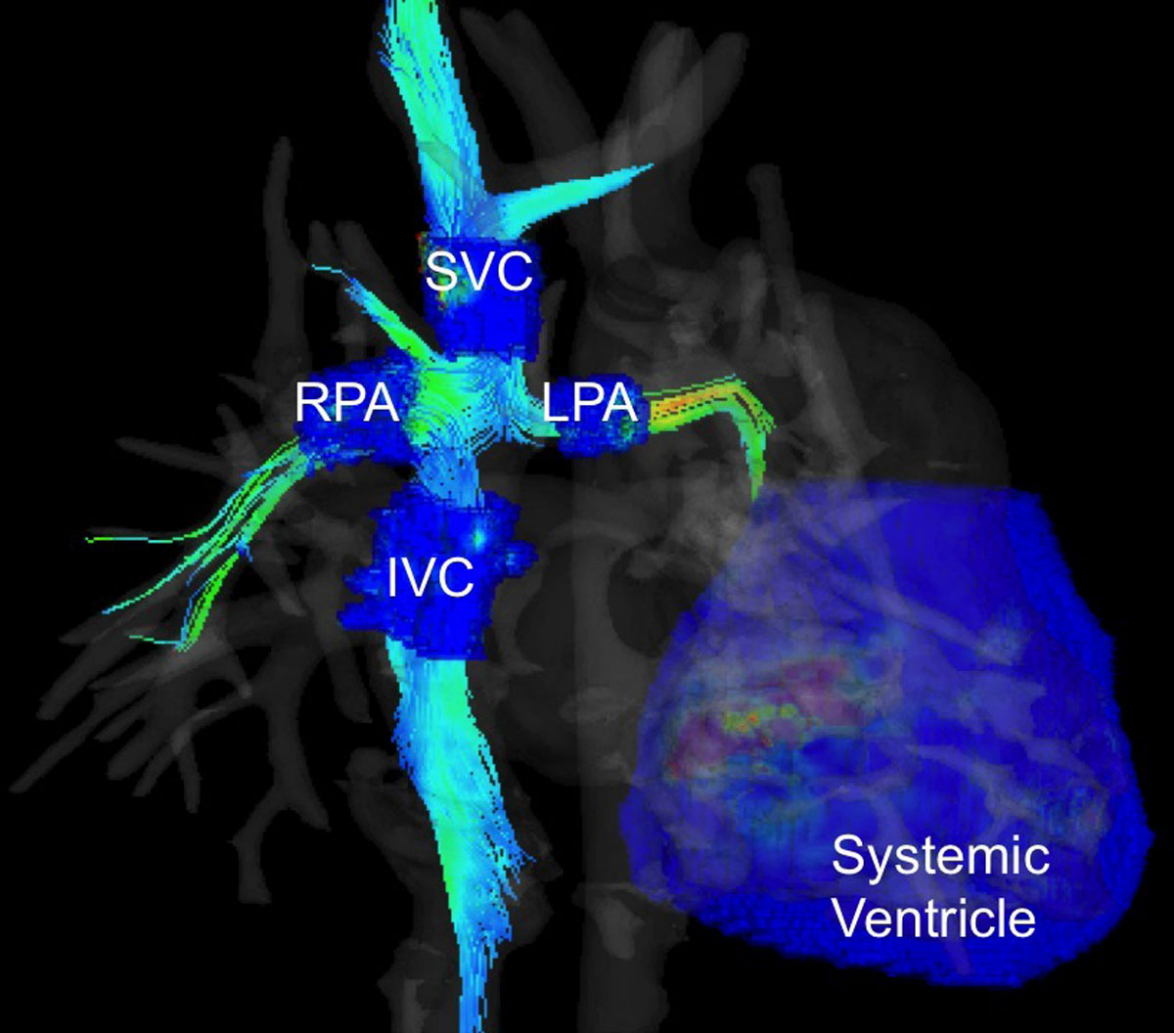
Visualization of flow and kinetic energy in an extracardiac TCPC and its systemic ventricle obtained from 4D flow MRI.
